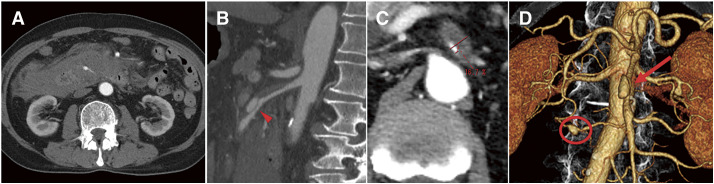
Contrast-enhanced computed tomography on initial presentation. (A) Axial view: Hemorrhagic ascites is noted around the pancreas. (B) Sagittal view (arterial phase): A false lumen-type dissection is observed at the origin of the superior mesenteric artery, measuring 5.5 cm in length, with narrowing of the true lumen (arrowhead). (C) Axial view of the SMA stenosis: The degree of SMA stenosis was calculated to be 83.3%. The percentages indicate the ratio of the true lumen to the overall vascular diameter. (D) Three-dimensional reconstruction of the abdominal artery: Dissection of the superior mesenteric artery is visualized (arrow). An aneurysm measuring 12.1 × 8.4 mm is identified in the anterior superior pancreaticoduodenal artery (circle), considered the source of bleeding. SMA: superior mesenteric artery
